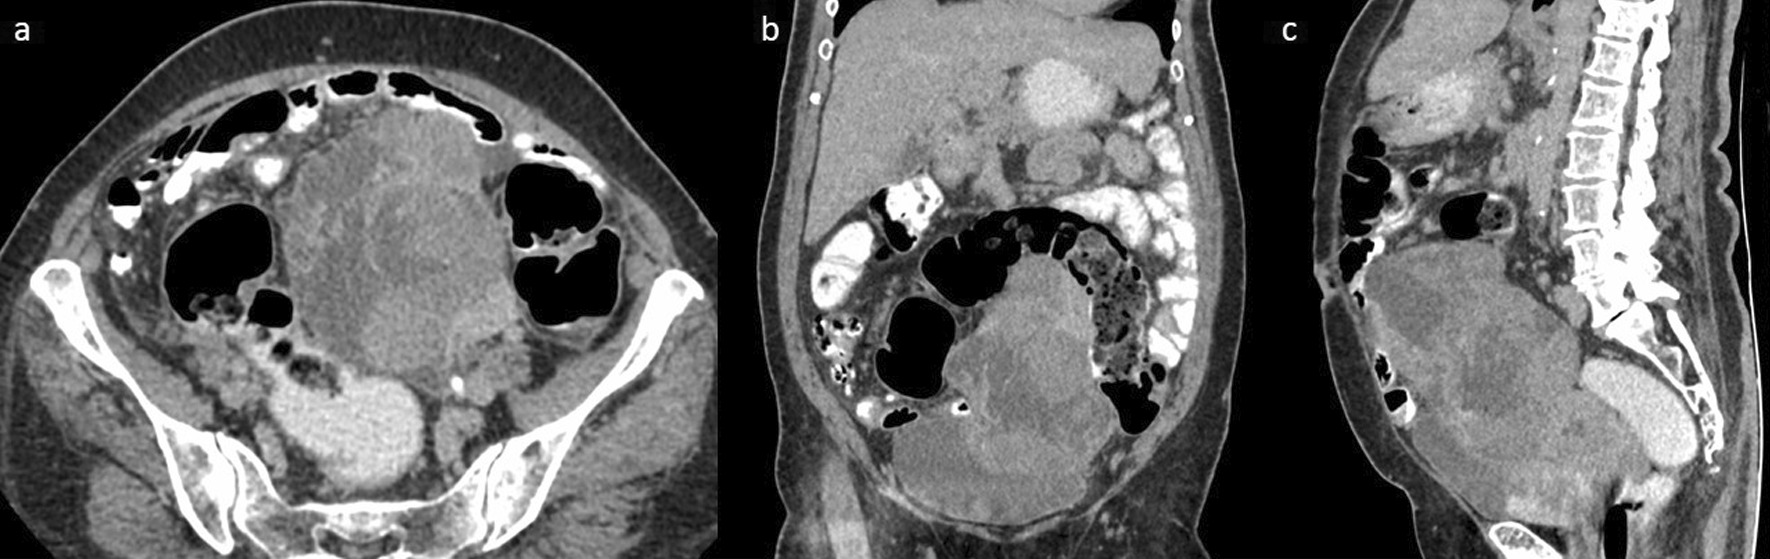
Contrast-enhanced CT of the abdomen and pelvis showing a heterogeneously enhancing complex mass arising from the left adnexal region. In the axial image (a), it measures 11 × 8 cm. There is no calcification, fat content, or air-fluid level within. In the coronal (b) and sagittal (c) images, it extends up to the level of the umbilicus abutting the anterior abdominal wall. Mass merges with uterus and bladder with no intervening fat planes. The mass displaces the small and large bowel
